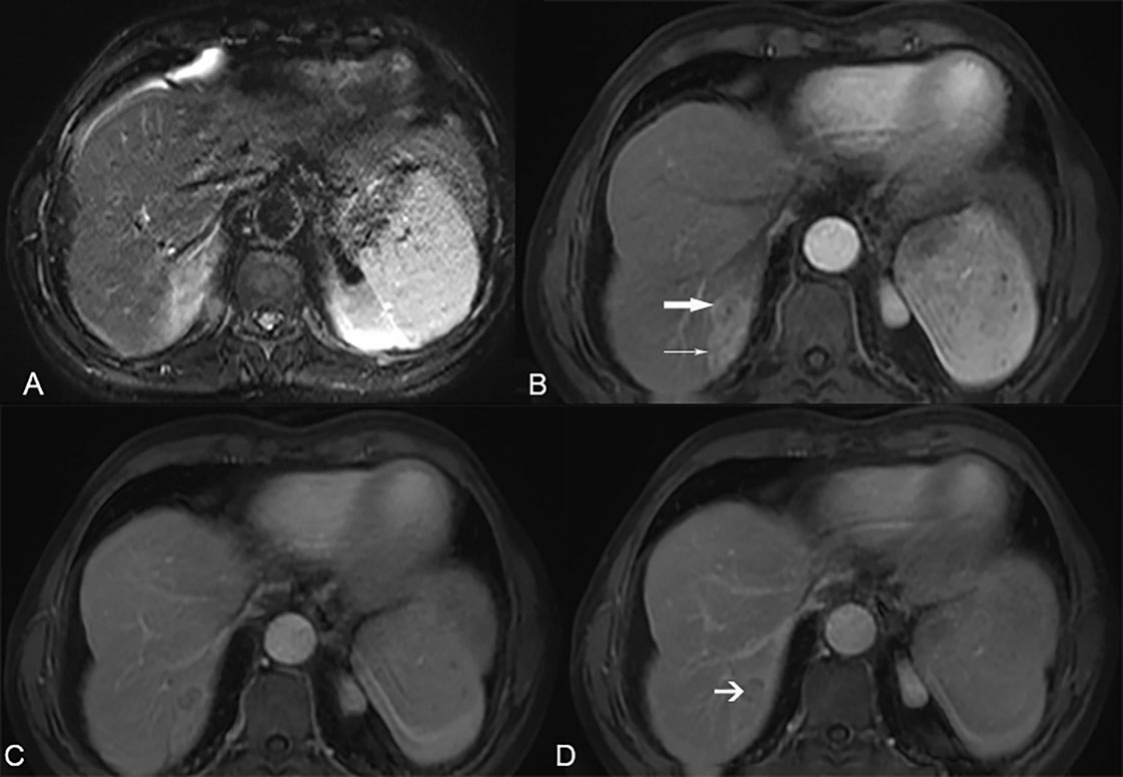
A 61-year-old male patient with hepatitis B-related liver cirrhosis and cHCC-CCA is categorized as LR-M category. (A) T2-weighted image shows a 36-mm nodule with heterogeneous hyperintensity in hepatic segment VIII. (B) The arterial phase shows peripheral enhancement (arrow) and tumor thrombus in the bile duct (thin arrow) on contrast-enhanced T1-weighted imaging with the contrast agent Gd-DTPA. (C) The portal venous phase and (D) delay phase show targetoid appearance and the arrow shows progressively delayed central enhancement.
